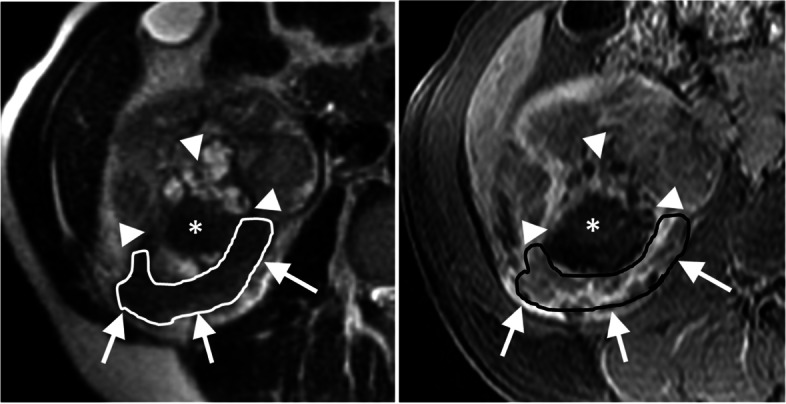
58-year-old man with sarcomatoid RCC showing small volume of T2LIA. T2-weighted axial image (A) and post-contrast T1-weighted image (B) shows 11.7-cm right kidney mass demonstrating small area T2LIA (arrows; volume of 15.2 mL; 2.5%) and adjacent non-enhancing area (arrowhead), probably representing hemorrhage (*) and/or necrosis. In the covered lung base, a 3.6-cm left lower lobe lung metastasis was noted (not shown). Cytoreductive radical nephrectomy was done after which pathological analysis showed a 11.5-cm clear cell RCC with extensive sarcomatoid differentiation and renal sinus fat invasion. Systemic treatment including sunitinib and gefitinib was started owing to progression of disease at the thoracic and abdominal lymph nodes, chest wall and lungs. Patient died 226 days after surgery. RCC = renal cell carcinoma; T2LIA = T2 low signal intensity area
